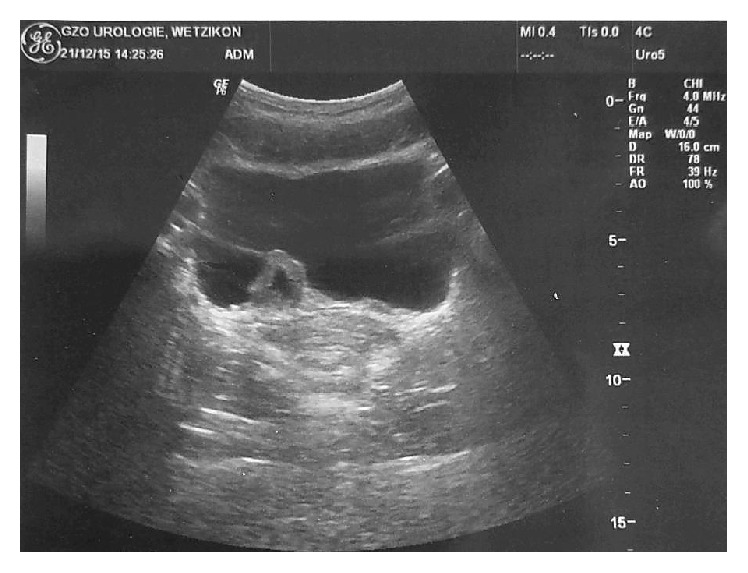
Transversal section of the sonographic control at the 12-month follow-up. The left-sided ureterocele can be seen side-inverted on the picture's right side. Compared to the initial CT scan (Figure 3), there is a significant regression of the ureterocele in size.
